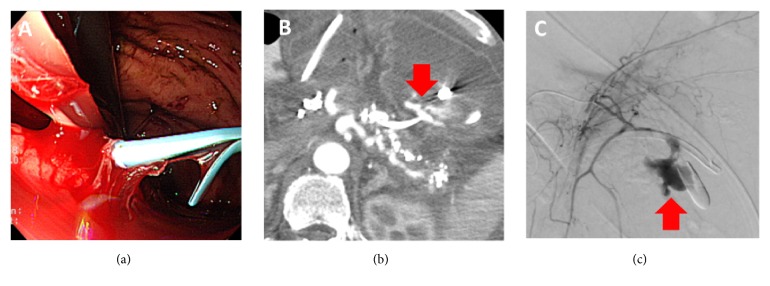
Bleeding occurred at the 371st day after EUS-PD in case 3. (a) Arterial bleeding from the transgastric puncture tract. (b) Contrast-enhanced computed tomography revealed extravasation into the stomach (arrow). (c) Interventional radiology revealed a pseudoaneurysm from the left gastric artery (arrow), and arterial embolization was performed.
